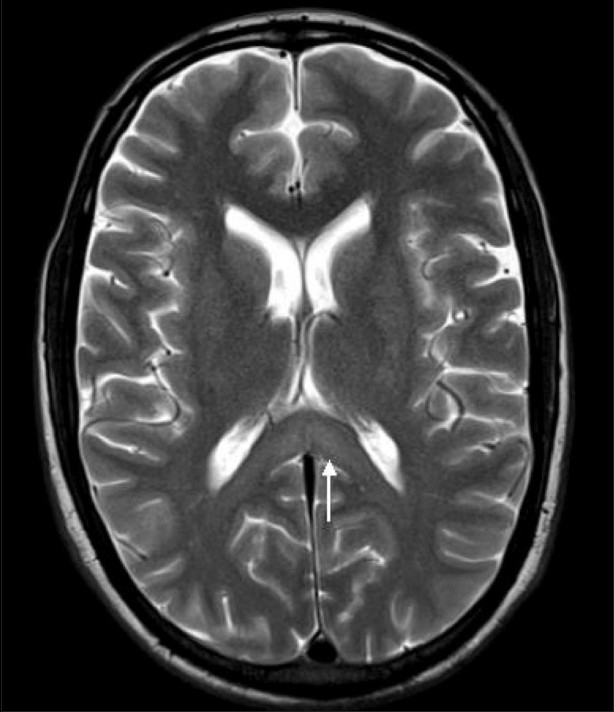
Axial T2W MRI of the brain shows symmetric areas of hyperintensity involving the splenium of the corpus callosum (arrow)
